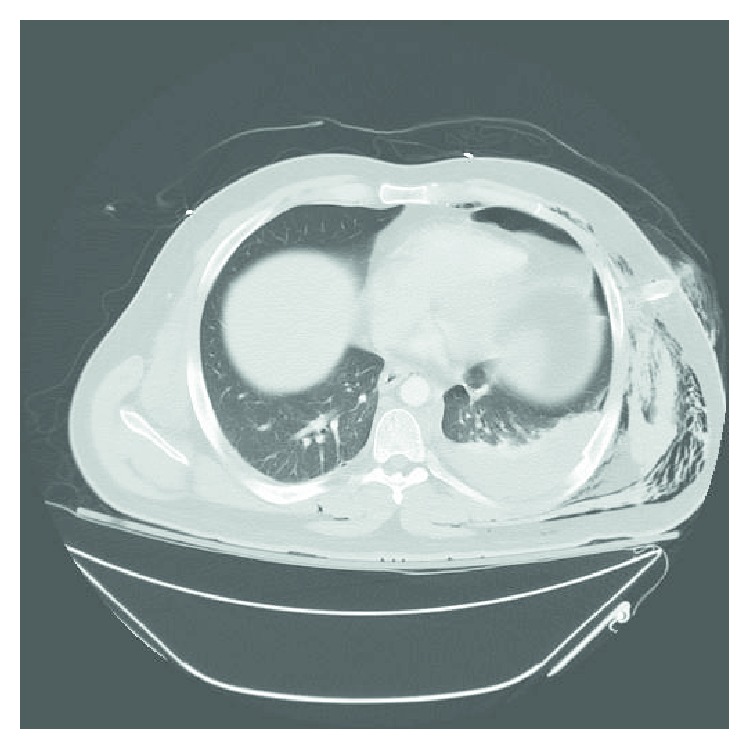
Computed tomography of the chest from January 2012 showing left-sided hemothorax and subcutaneous emphysema.
